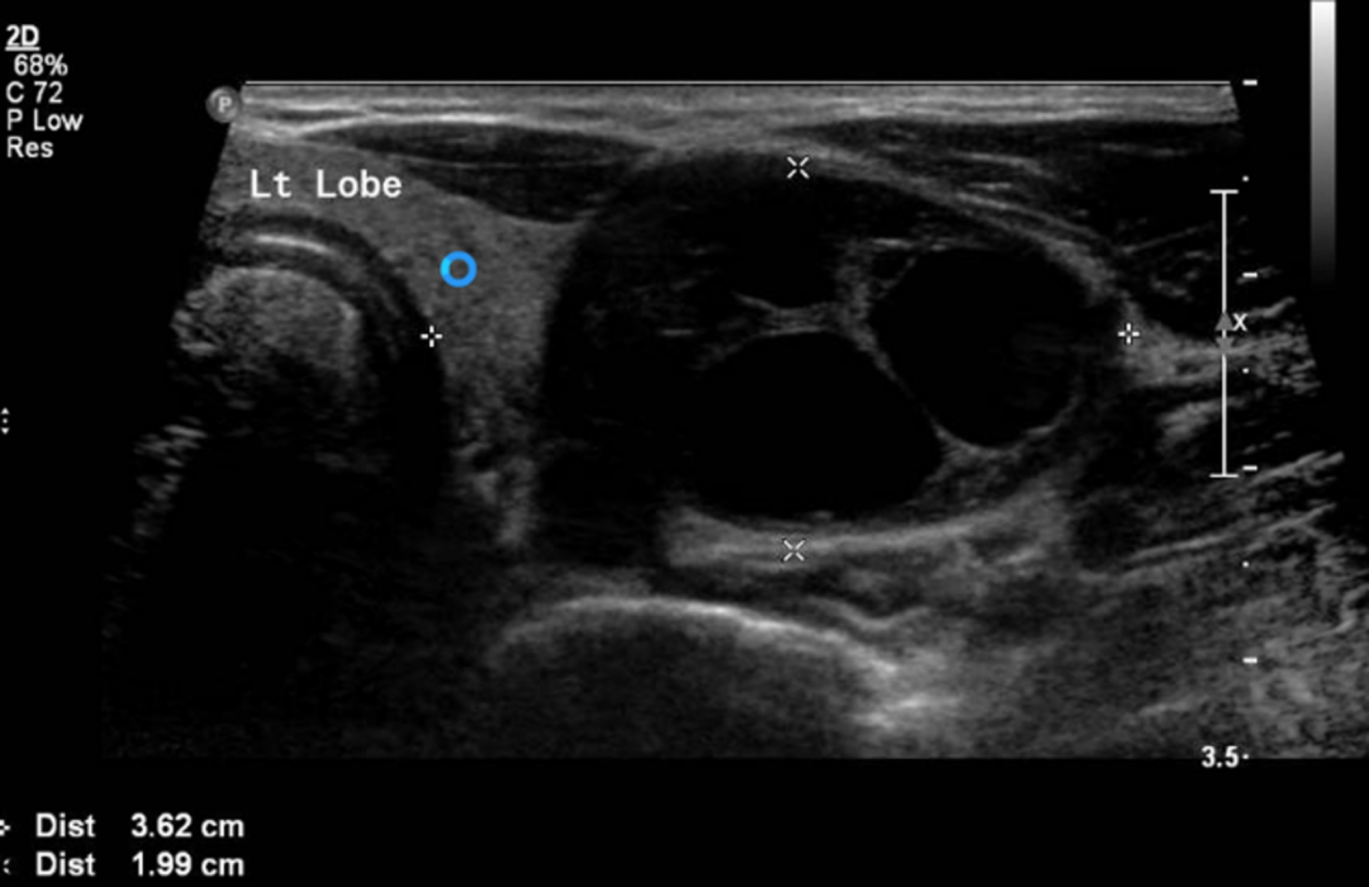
Ultrasonography of the thyroid gland revealing left lobe of 3.6 x 2.0 cm in size with a large heterogeneous, predominantly cystic, nodule measuring about 7.5 x 2.4 cm in the longest dimensions
